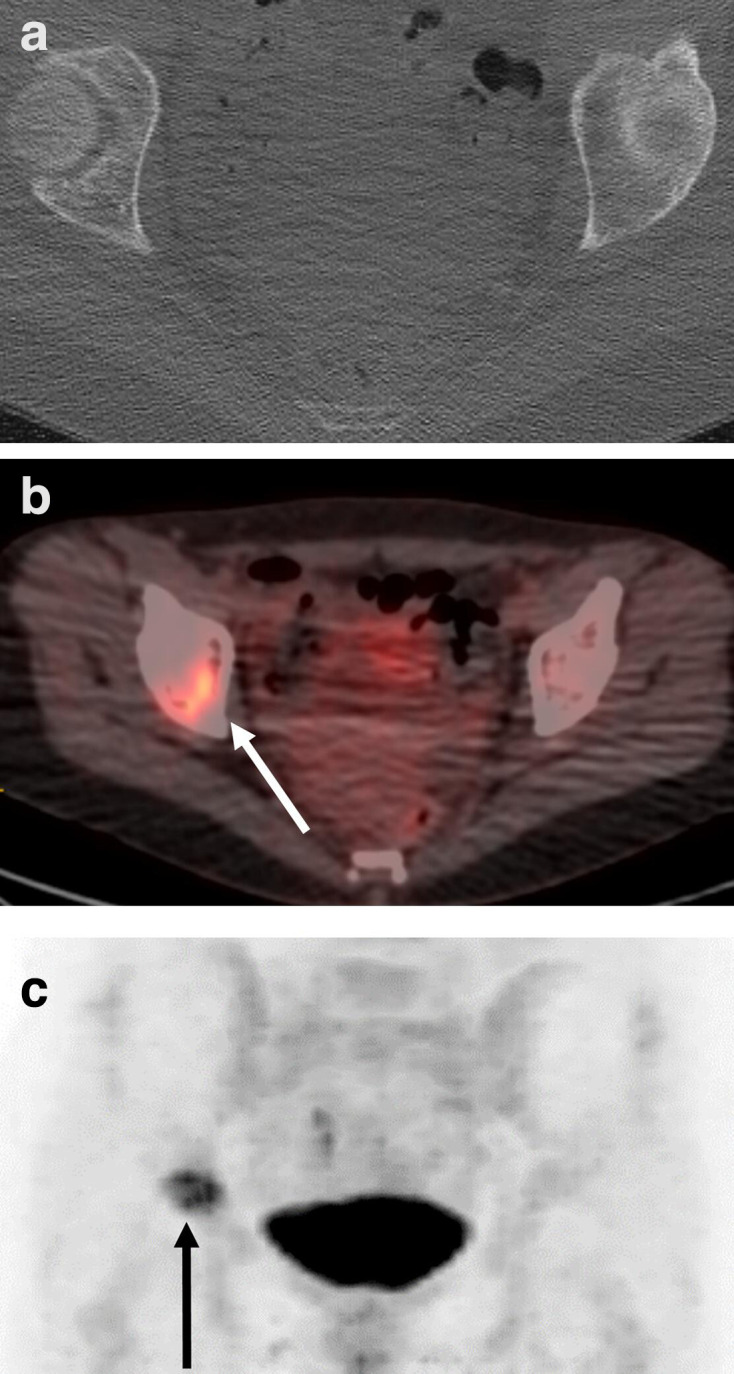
Axial contrast-enhanced FDG-PET/CT of a 59-year-old female patient with NSCLC. The bone metastasis in the right acetabulum was not visible on the (a) CT, while it was clearly visible on the (b, c) FDG-PET (arrow). The accuracy of the FDG-PET/CT to detect bone metastasis is higher compared to CT alone. FDG, fludeoxyglucose;PET, positron emission tomography.
